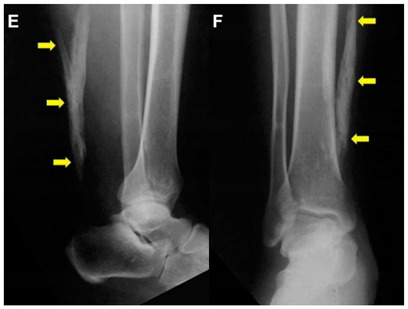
Romano et al., 2020 ^d^ [11]	A 58-year-old woman. This image submission includes a lateral view X-ray (Image G), ultrasound (Image H), MRI (Image I), and STIR image (Image J). In image G the asterisk represents the gap separating the two ossifications (arrows) within the Achilles Tendon. Image H demonstrates a hyperechoic gap (asterisk) between tendon ends (arrows) which is suggestive of a complete rupture of the ossification. Images I and J demonstrate hemorrhage and edema (asterisk) filling the space between the separated ends of the Achilles Tendon. The patient had a palpable gap on examination of the Achilles Tendon. The images confirmed the diagnosis of a complete rupture of ossified tendon. The patient had no history of trauma or surgery in the area. The patient was managed with immobilization in a plaster cast. The patient’s recovery status was not reported.
